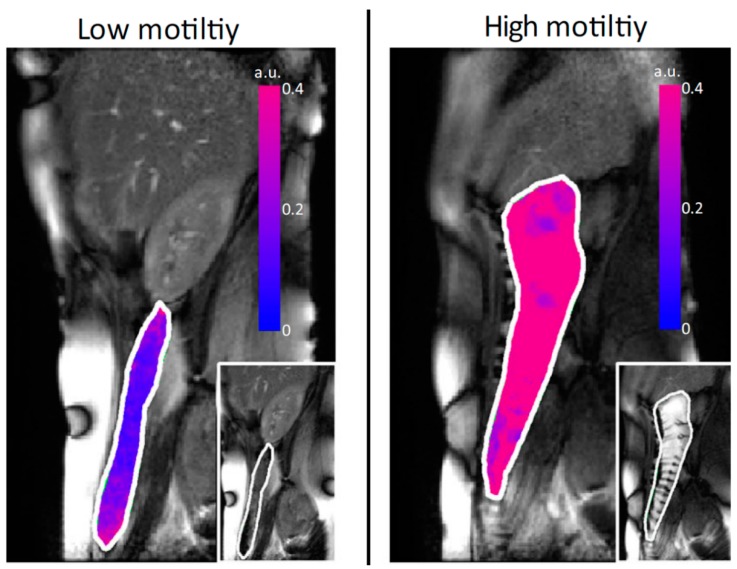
Motility assessment by the cine MRI technique. The figure depicts overlay (red/blue) of standard deviation of Jacobian. Reproduced from [28], Blackwell Publishing LTD, 2015 with permission.
